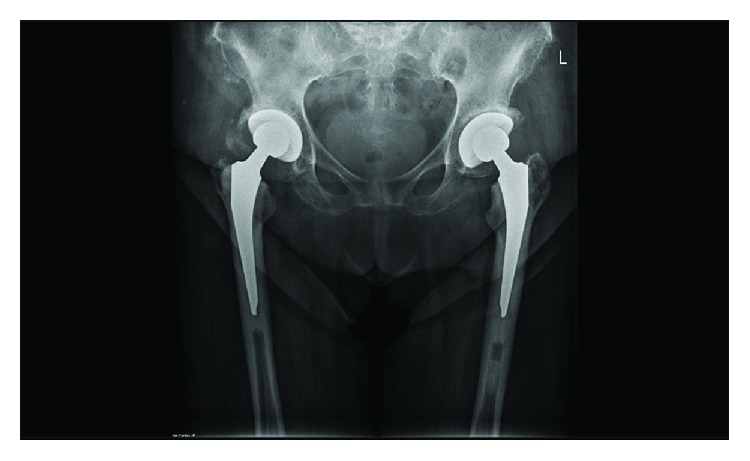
8 weeks of follow-up anteroposterior X-ray of the pelvis.
